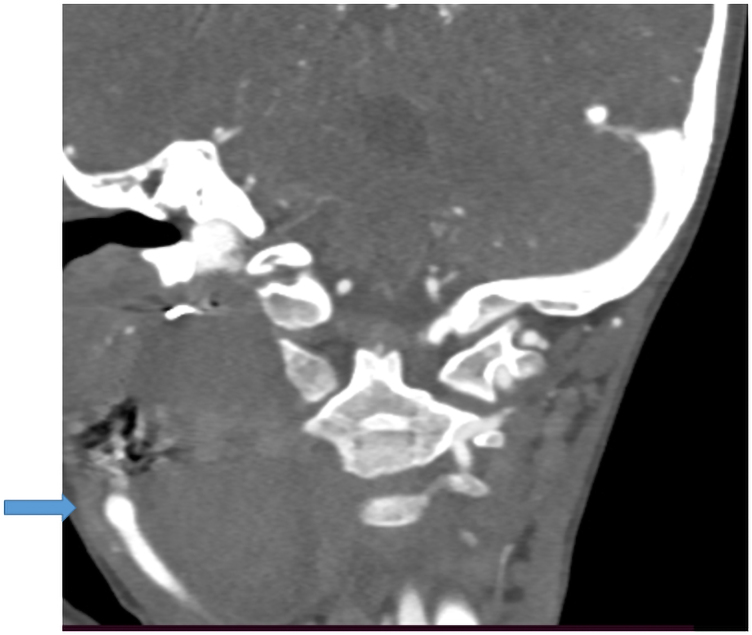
Postoperative CT angiogram (day 4) showing no residual aneurysmal filling and a resolving parapharyngeal haematoma.
